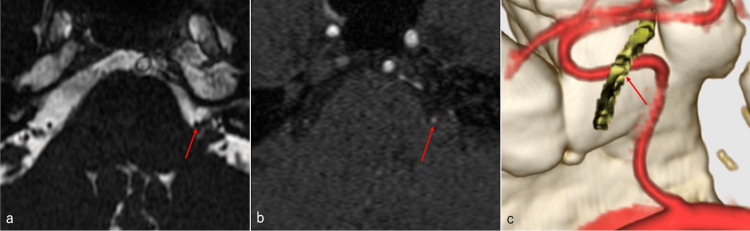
Left anterior inferior cerebellar artery in contact with the distal portion of the left facial nerve The left anterior inferior cerebellar artery was found to be in contact with the distal portion of the left facial nerve, but no responsible vessel could be noted in the root exit zone of the left facial nerve. a) Head magnetic resonance imaging (fast imaging employing steady-state acquisition); b) Head magnetic resonance angiography original image; c) 3D structured image. Red arrow: Distal facial nerve compression site by the left anterior inferior cerebellar artery.
